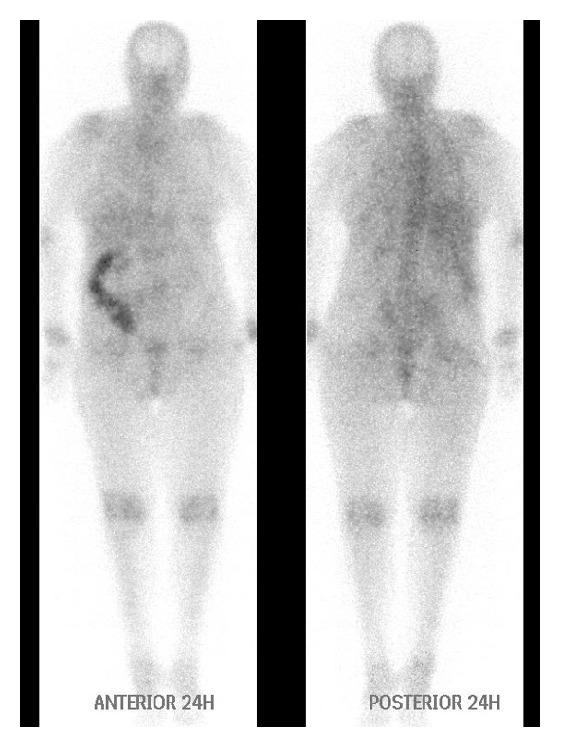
The gallium-67 scintigraphy revealed persistent diffuse concentration of the gallium in the ascending colon at 24-hour imaging. Gallium-67 excreted into the bowel and laxatives are essential for the differential diagnosis between true positive increased bowel activity and false positive uptake due to bowel content.
